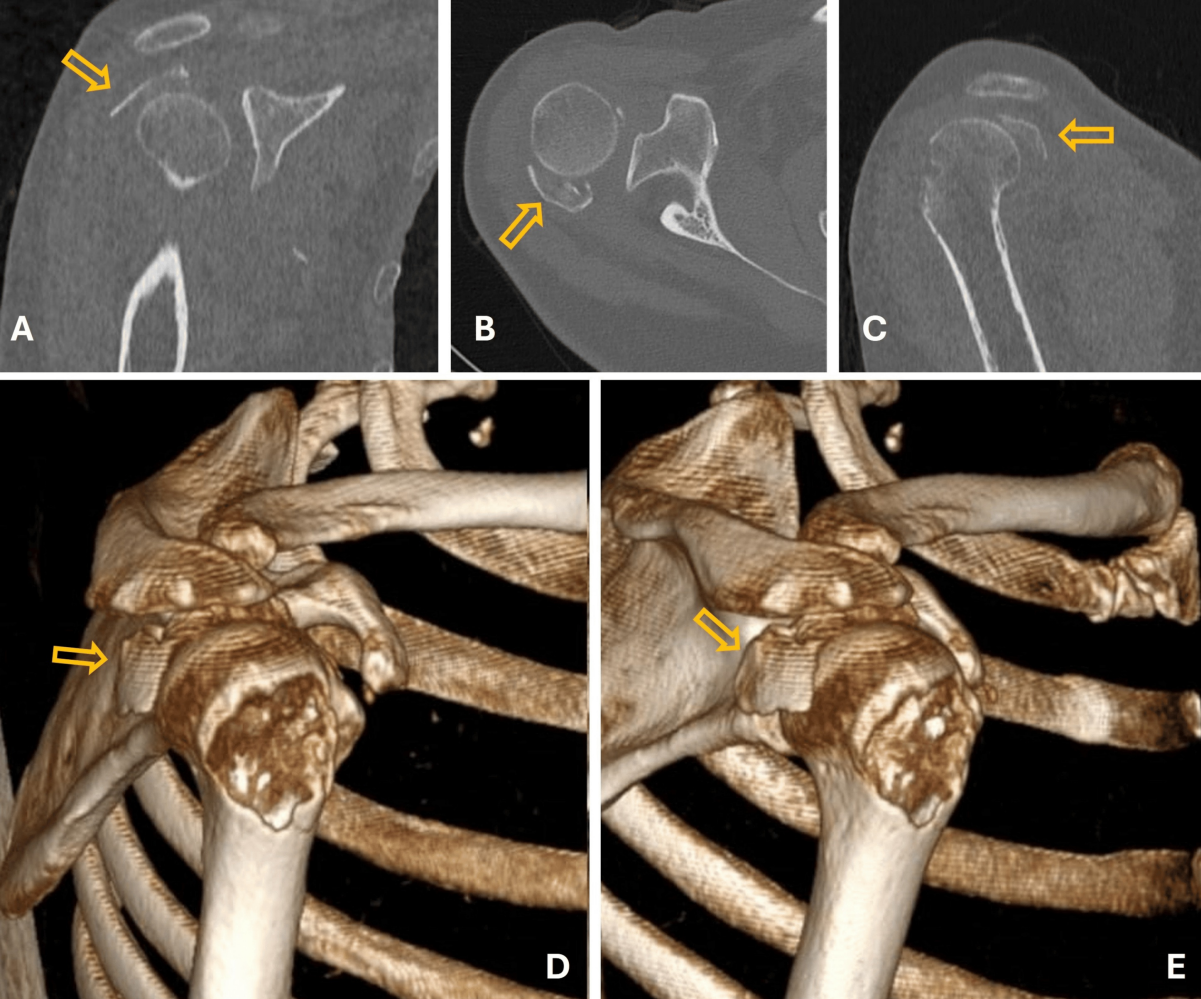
Computed Tomography (CT) and 3D Reconstruction Demonstrating Displaced Greater Tuberosity Fragment of the Right Shoulder Coronal (A), axial (B), and sagittal (C) CT images, along with three-dimensional reconstructions (D, E), demonstrate the displaced greater tuberosity fragment of the right shoulder (yellow arrows).
